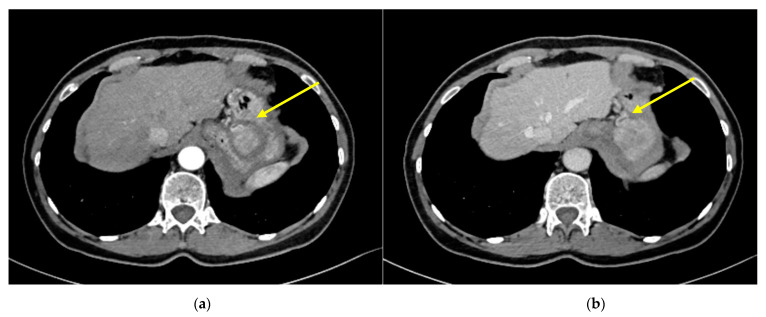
Axial contrast-enhanced computed-tomography (CT)-scan images: (a) arterial phase; (b) portal phase. Arrow: ovaloid-shaped solid mass.
